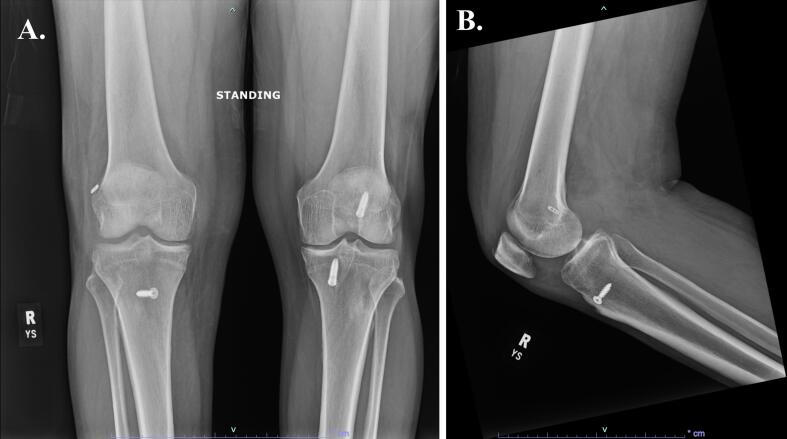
Radiographs of the right knee demonstrating (A) bilateral standing AP and (B) lateral.
